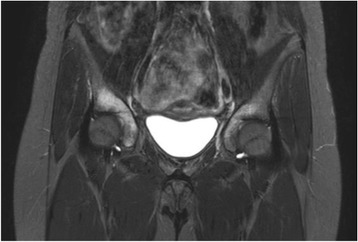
Case 1: Imaging data of CNO involving pelvis in a 12-year-old girl. Coronal STIR MRI reveal increased signal in the right and left acetabulum
